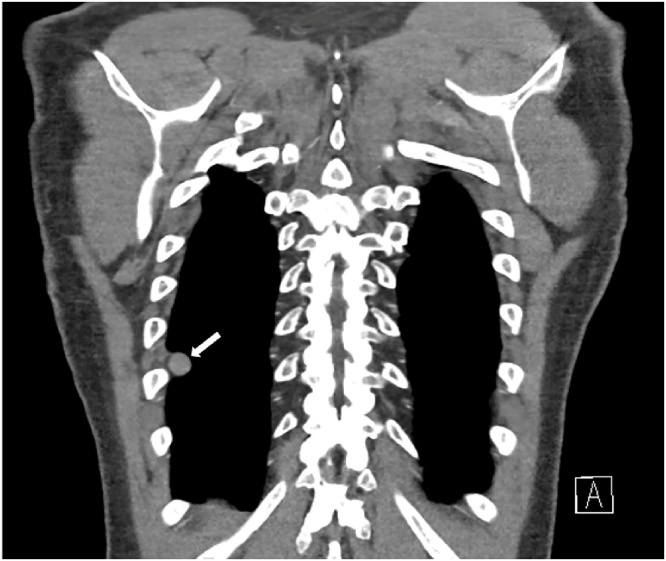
CT demonstrating isolated right pleural metastasis (white arrow).
